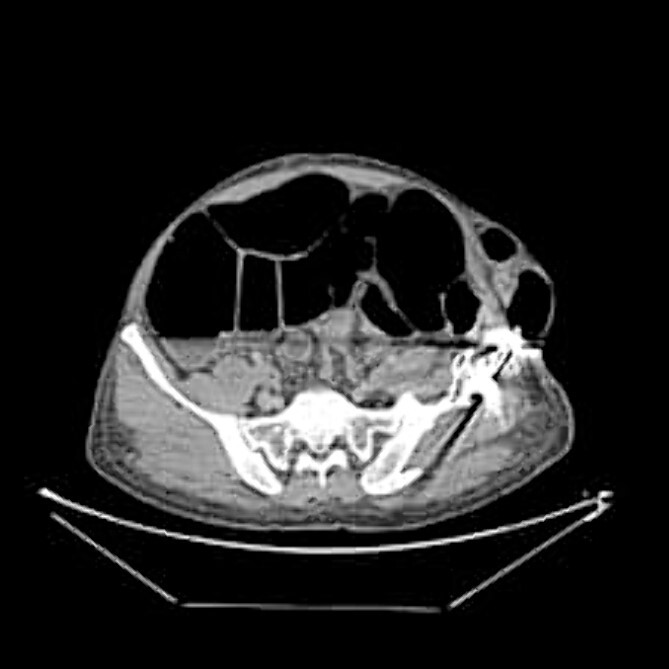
Axial abdominal CT scan.
